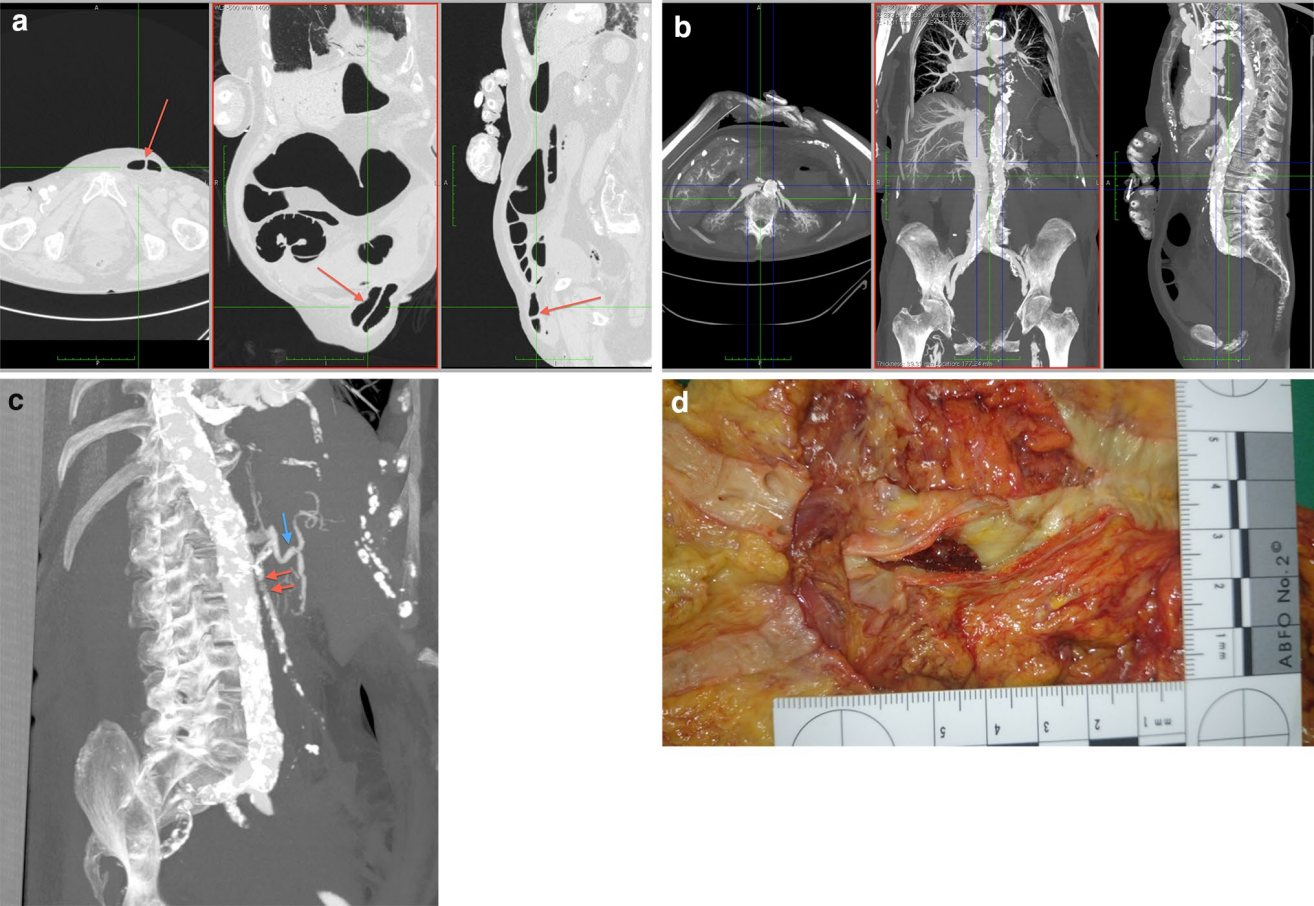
a–d A 94-year-old female patient was admitted to hospital with epigastric pain and ground vomiting the day before. *Diagnosis on admission* leftside inguinal hernia, no convincing indication for ileus/acute abdomen. While the consiliary surgeon decided to wait and see, laboratory markers indicated myocardial ischemia. On the second day after admission, a transesophageal echocardiography was performed with propofol sedation. 2 h later the patient was found dead on the ward with apparent preceding fecal vomiting. a Dilated intestine with inguinal hernia (*red arrow*) native PMCT, MPR; b MPMCTA, dynamic phase, MIP. Severe overall calcification of the aorta. Inferior vena cava and liver venes. Note the missing contrast enhancement of the mesenteric vasculature; c MPMCTA, arterial phase, MIP. Aorta with celiac artery and common hepatic artery (*blue arrow*). Superior mesenteric artery with severe sclerosis, proximal faint opacification (*red arrows*), ending without visualization of major branches. Inferior mesenteric artery missing either. d Fresh thrombosis of proximal mesenteric artery (autopsy finding). The patient died of paralytic ileus due to mesenteric infarction which had been accompanied by cardiac failure with myocardial ischemia
